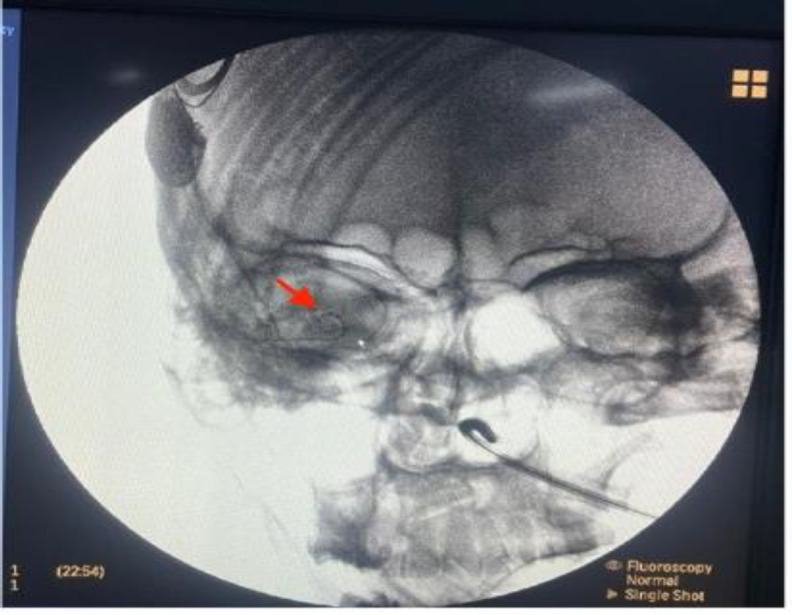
Post cochlear implantation C Arm generated X-ray showing the implant on right side (red arrow)
